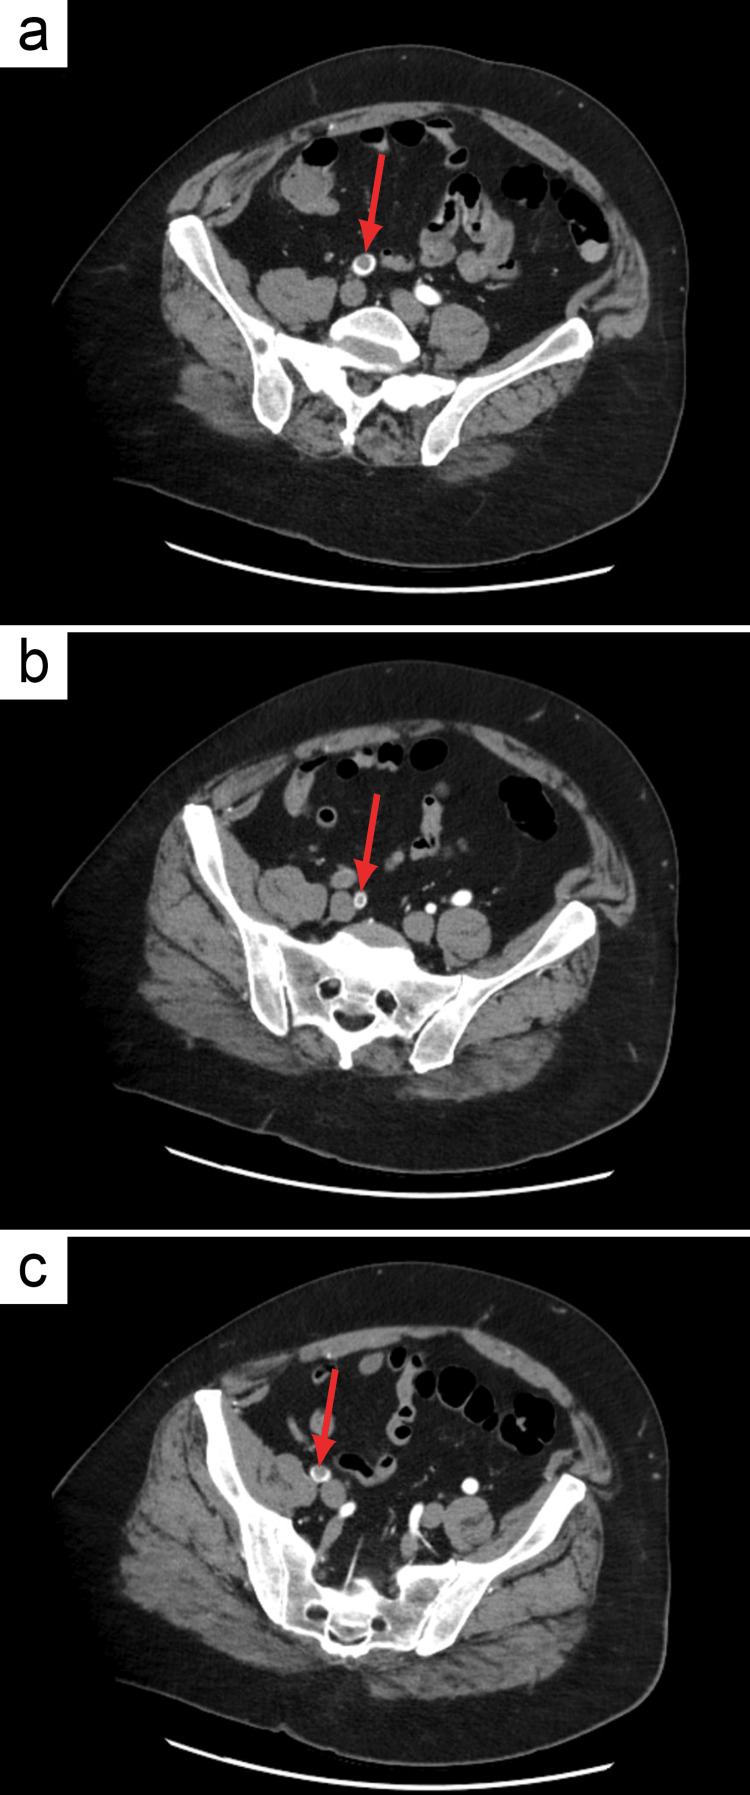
Axial computed tomography angiography of the patient’s abdomen/pelvis. The repeat chest, abdomen, pelvis, and run-off computed tomography angiography confirming acute limb ischemia findings, including thrombus (red arrows) within the iliac vessels. (a) Non-occlusive thrombus in the right common iliac artery. (b) Non-occlusive thrombus in the right internal iliac artery. (c) Occlusive thrombus in the proximal right external iliac artery.
